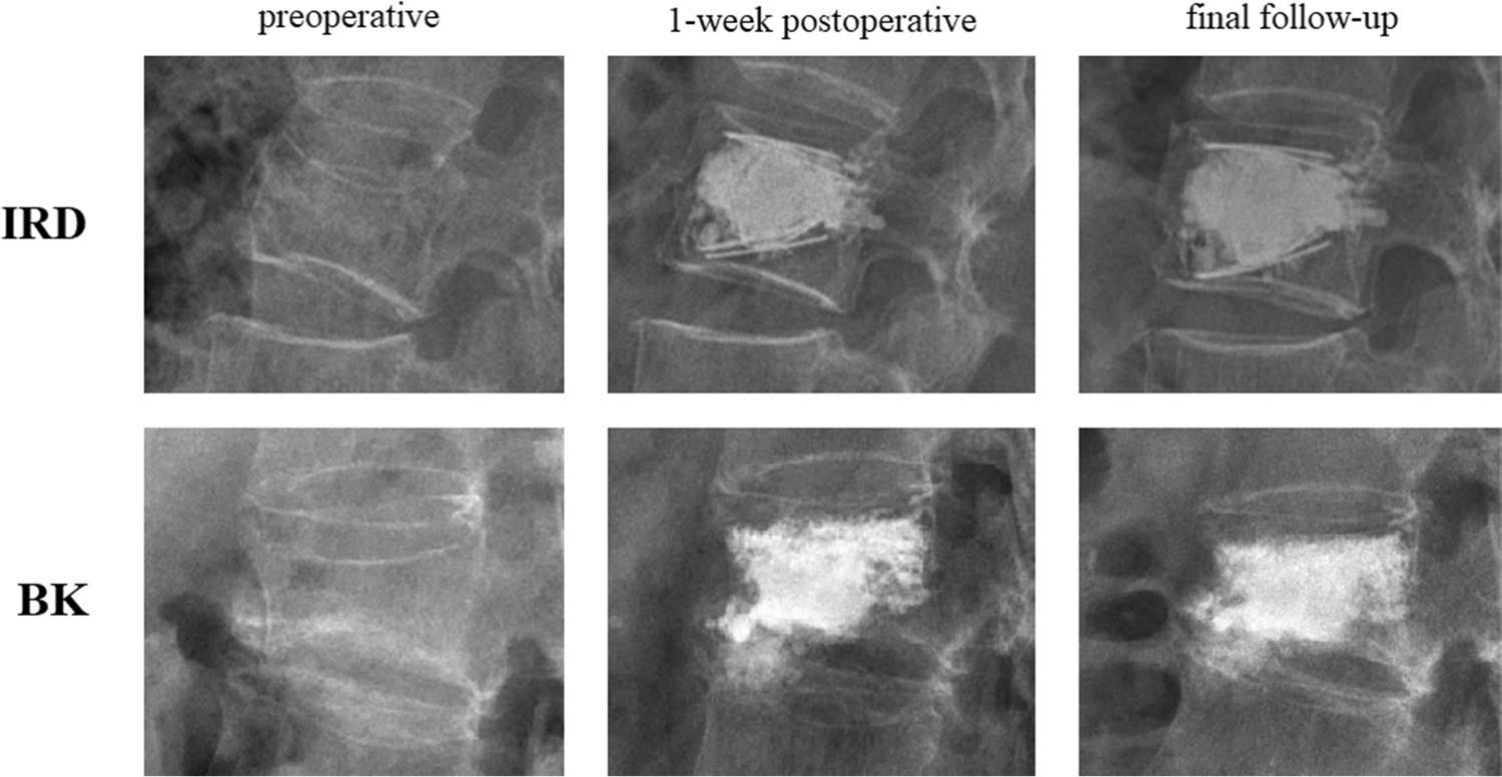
Radiographic demonstration of KP with IRD and BK: preoperative, 1-week postoperative, and final, respectively. (*KP with IRD* kyphoplasty with intravertebral reduction device, *BK* kyphoplasty with balloon).
